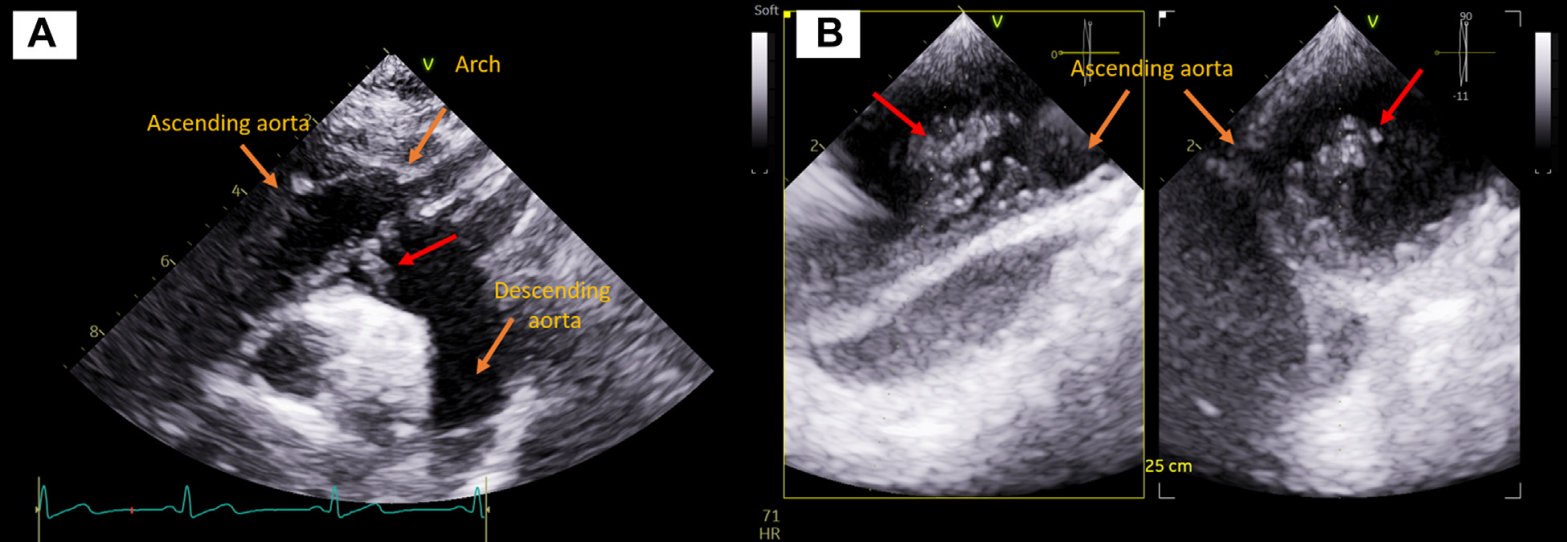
TTE and TOE Images (A) Transthoracic echocardiography (TTE) image shows the principal structures such as the arch, ascending aorta, and descending aorta indicated by orange arrows, while the lesion with irregular margins and distal appendages is indicated by the red arrow. (B) Transesophageal echocardiography (TOE) image shows the lesion at 25 cm from the buccal rim in the ascending aorta, indicated by the red arrow.
